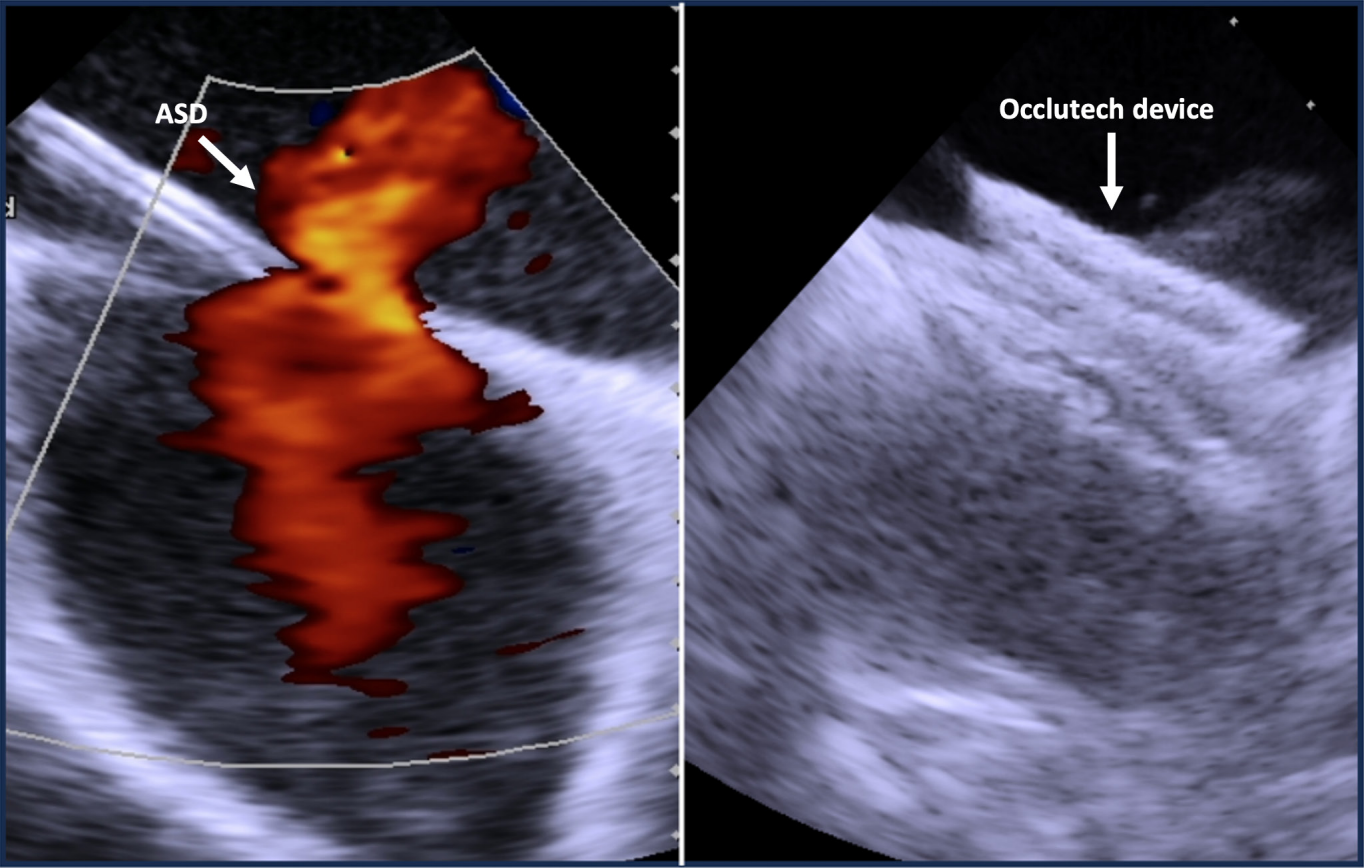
Intracardiac ultrasound evaluation of atrial septal defect and percutaneous closure with Occlutech 27‐mm.
